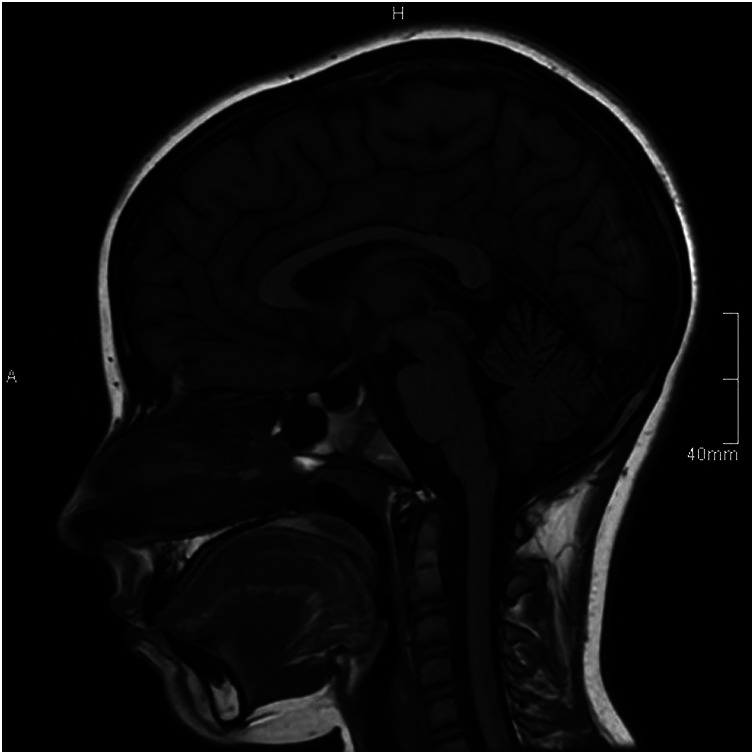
Sagittal T1 magnetic resonance imaging showing a partially empty sella filled with cerebrospinal fluid with a thin rim of pituitary tissue.
